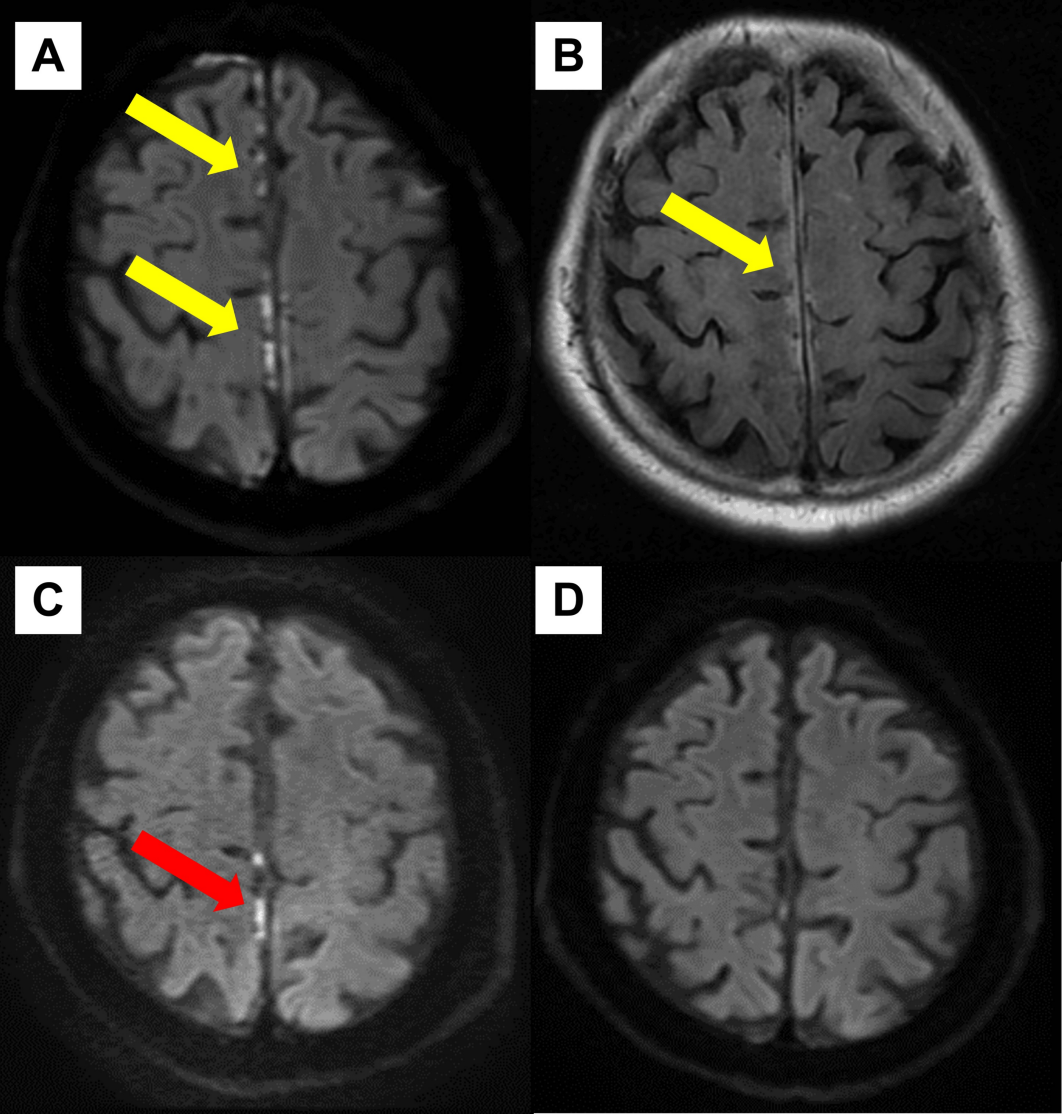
Serial changes in brain MRI findings. On day 1, brain MRI showed hyperintense signals on DWI and fluid-attenuated inversion recovery sequences along the meninges of the right-dominant bilateral frontoparietal lobes (yellow arrows). (A, B) Follow-up DWI-MRI on days 20 and 77 after treatment initiation revealed reduced hyperintensity along the meninges (red arrow) (C) and the disappearance of the findings (D), respectively. DWI: diffusion-weighted imaging, MRI: magnetic response imaging.
